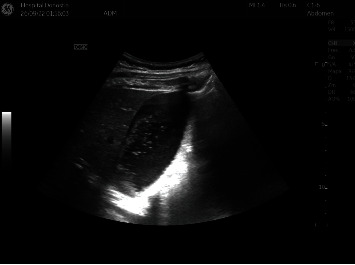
Abdominal ultrasound: distended gallbladder without clear ultrasound signs of acute cholecystitis and a minimal perihepatic fluid pocket were observed.
